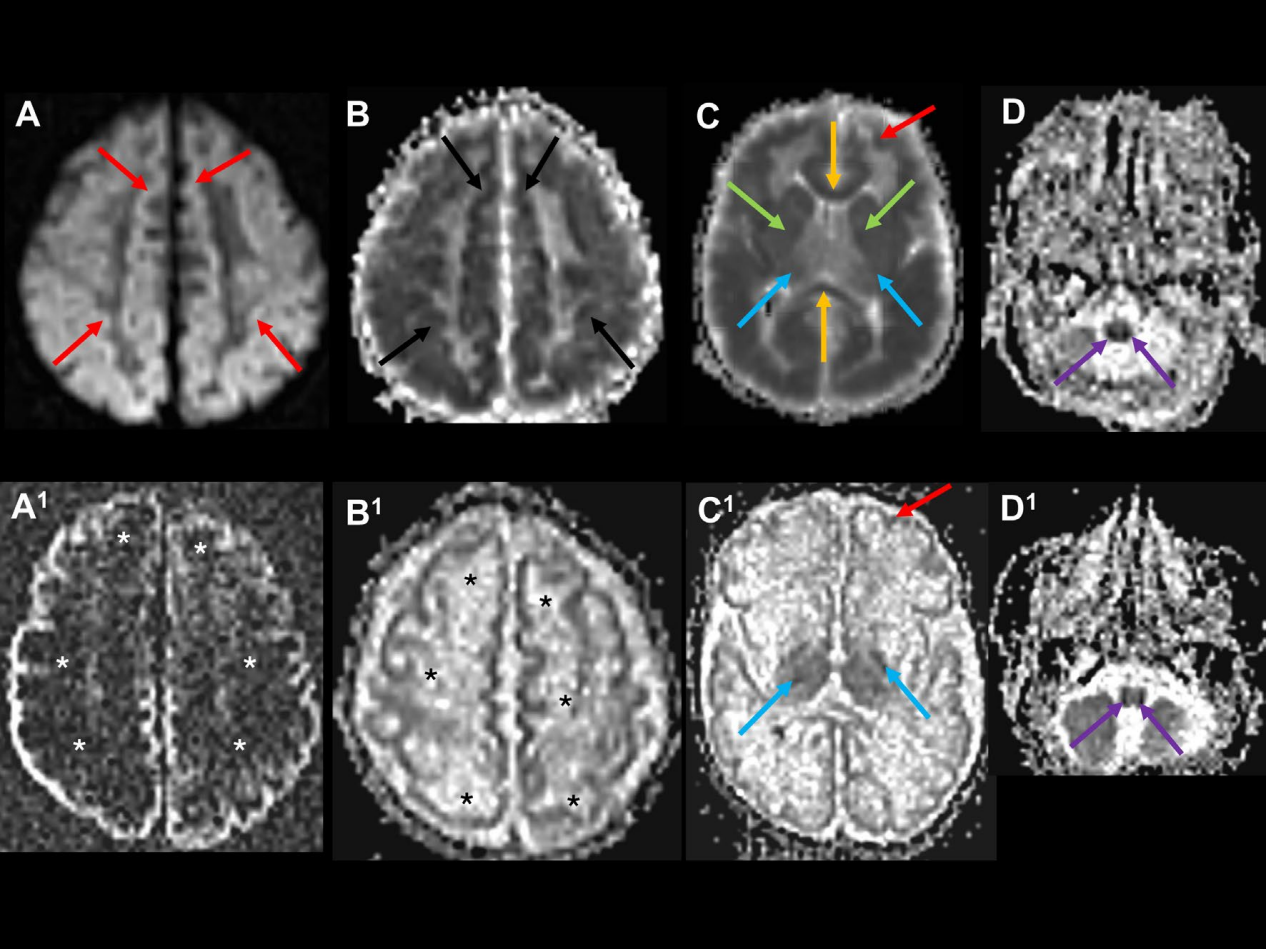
Persistence of cytotoxic edema in a patient with MoCD‐A treated with cPMP after onset of brain injury (Patient E). Upper row (A–D): At age 5 days; Bottom row (A1–D1): At age 20 days. A–B, A1–B1: DWI/ADC map at the centrum semiovale level. C–C1: ADC map at gangliocapsular level. D–D1: ADC map at the medulla oblongata level. A–B: Bilateral high DWI signal (red arrows) with corresponding low ADC (white arrows) demonstrates restricted diffusion in the frontoparietal cortex and subcortical white matter, indicating cytotoxic edema. Note, anterior frontal subcortical white matter is more affected than cortex on ADC map. A1–B1: Bilateral increased DWI/reduced ADC in the frontoparietal cortex indicates residual cytotoxic edema. Note resolved restricted diffusion in the subcortical white matter (asterisks). C: Bilateral low ADC signal/restricted diffusion indicates cytotoxic edema in the cortex and subcortical white matter (red arrow), callosal genu and splenium (orange arrows), basal ganglia (green arrows) and thalamo‐capsular region (blue arrows). C1: Bilateral low ADC signal indicates residual restricted diffusion in cortex (red arrow) and thalamo‐capsular regions (blue arrows). Note resolved restricted diffusion in subcortical white matter, corpus callosum, and basal ganglia. D: Bilateral low ADC signal/restricted diffusion indicates cytotoxic edema in the medulla oblongata (violet arrows). D1: Bilateral low ADC signal in the medulla oblongata is more discernible, indicative of ongoing cytotoxic edema in the medulla oblongata 16 days after treatment initiation (violet arrows).
